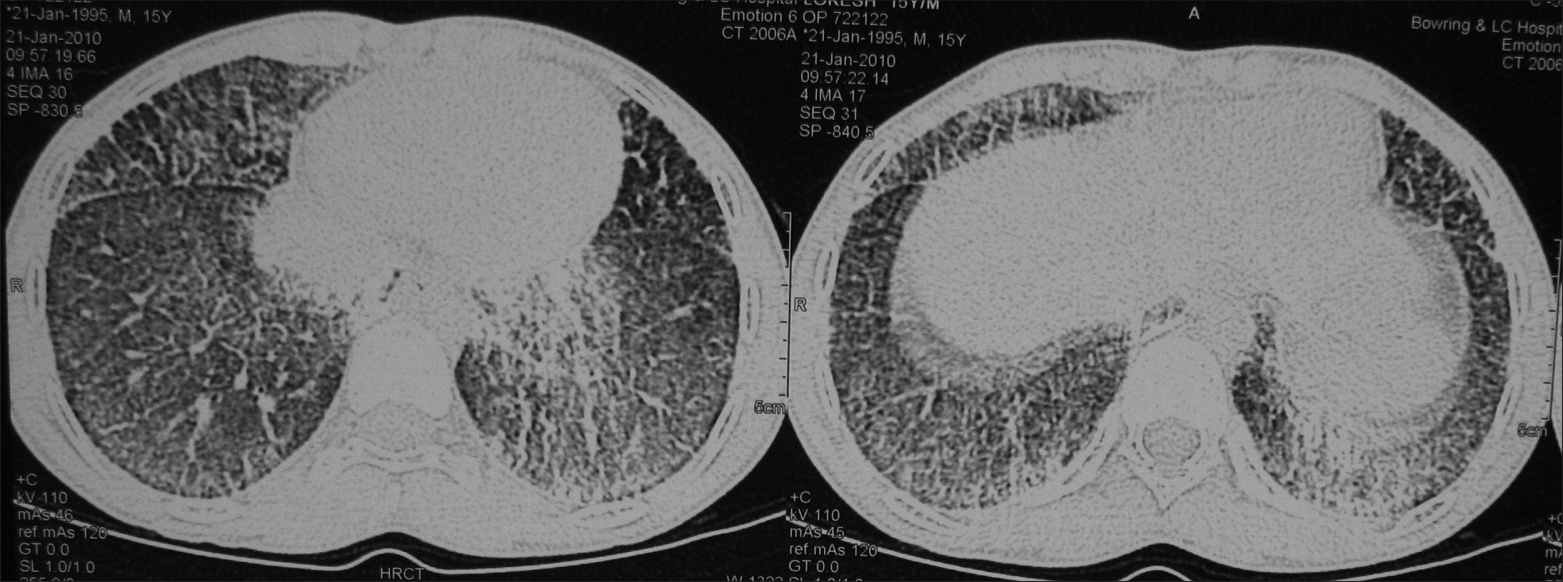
CT of Thorax showing bilateral diffuse micronodular opacities distributed predominanly over basal and posterior regions with reticular pattern
